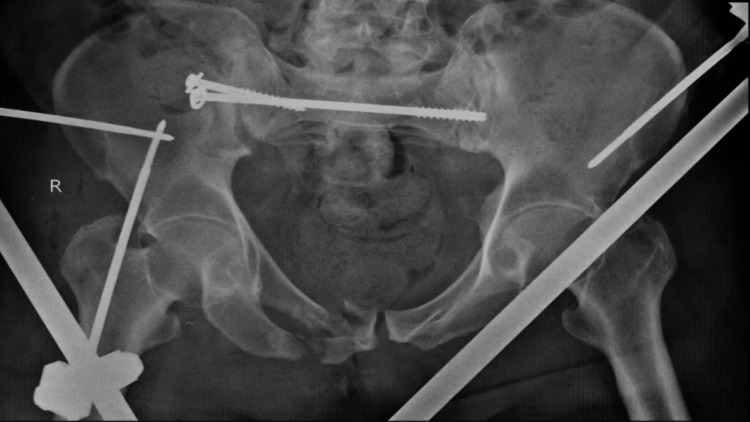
Postoperative x-ray showing the external fixator application for pelvis fracture and surgical correction of the right sacroiliac joint by CC screw. CC: Cannulated cancellous
